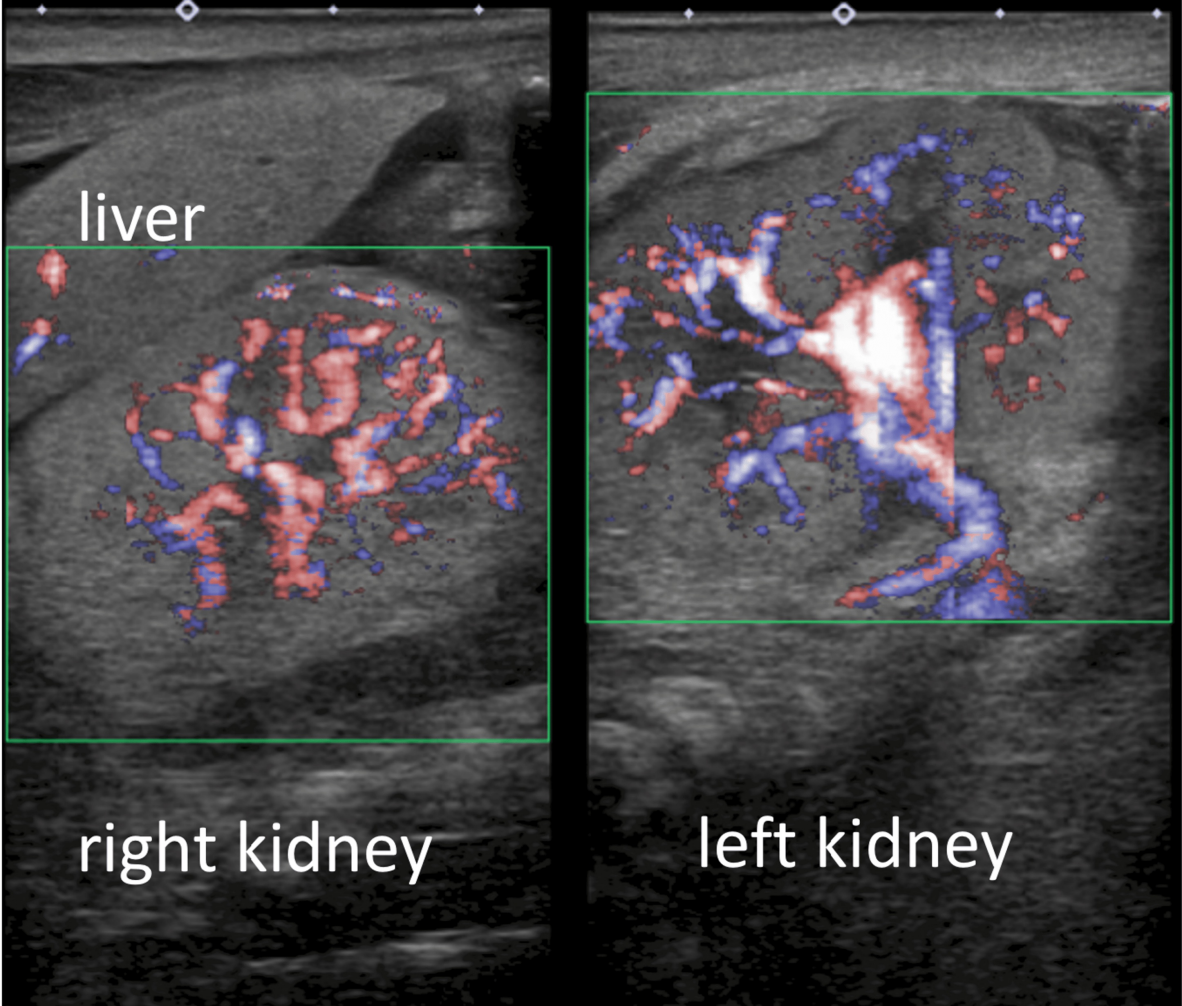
Color Doppler ultrasound on admission. The blood flow of both kidneys was normal.
